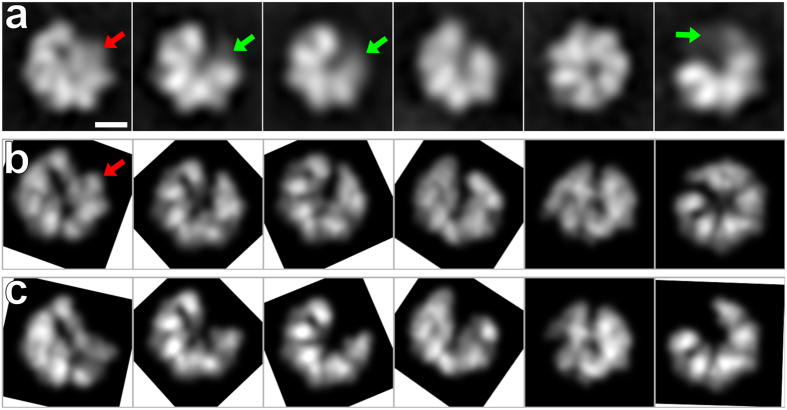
Structural heterogeneity of the shortened *h*LonΔ270 mutant. (a) 2D class averages of *h*LonΔ270 acquired by Relion show no sign of the N-terminal domain. Scale bar: 5 nm. Red arrows indicate features that could be uniquely assigned to a projection of a hexamer, green arrows point at decreased electron densities at the positions of the expected sixth 3M6A subunit. (b) Matching projections of six 3M6A subunits fitted into the AP-domain of the ADP-bound full-length Lon protein after the conversion of the x-ray structures to electron density maps. (c) Matching projections of five 3M6A subunits fitted into the AP-domain of the ADP-bound full-length Lon protein after the conversion of the x-ray structures to electron density maps.
